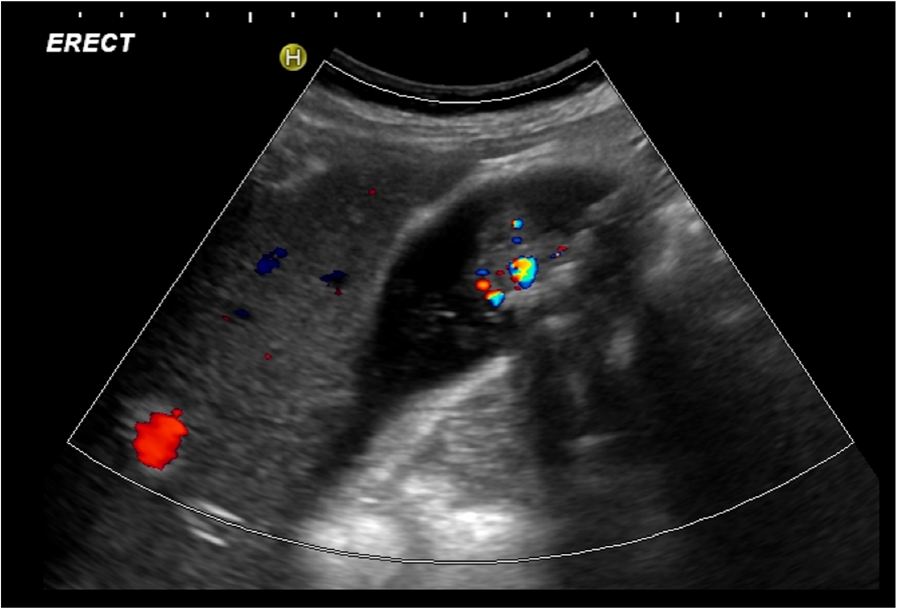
Sagittal ultrasound of the gallbladder demonstrating a soft tissue mass within the gallbladder with internal vascularity consistent with a gallbladder carcinoma
